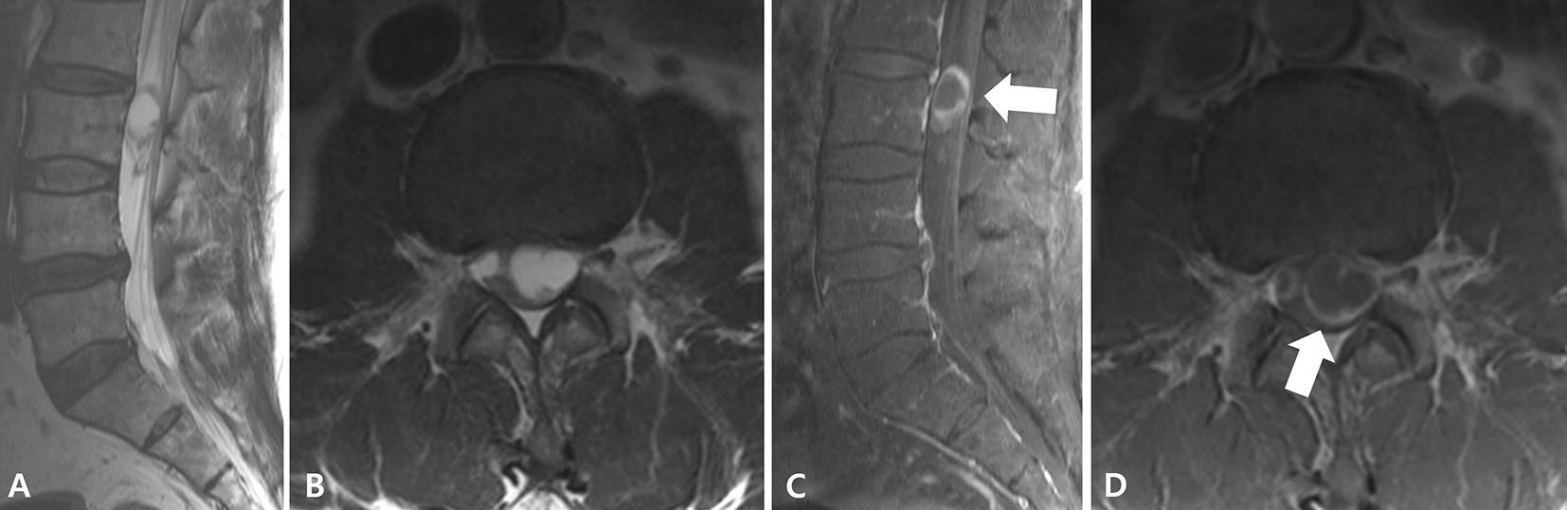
A schwannoma in a 59-year-old male patient at the L3 level. Sagittal (A) and axial (B) T2-weighted images show a 2.6 cm-sized intradural mass with hyperintense signal. Sagittal (C) and axial (D) fat-suppressed contrast-enhanced T1-weighted images demonstrate rim enhancement pattern (arrows) of the mass.
